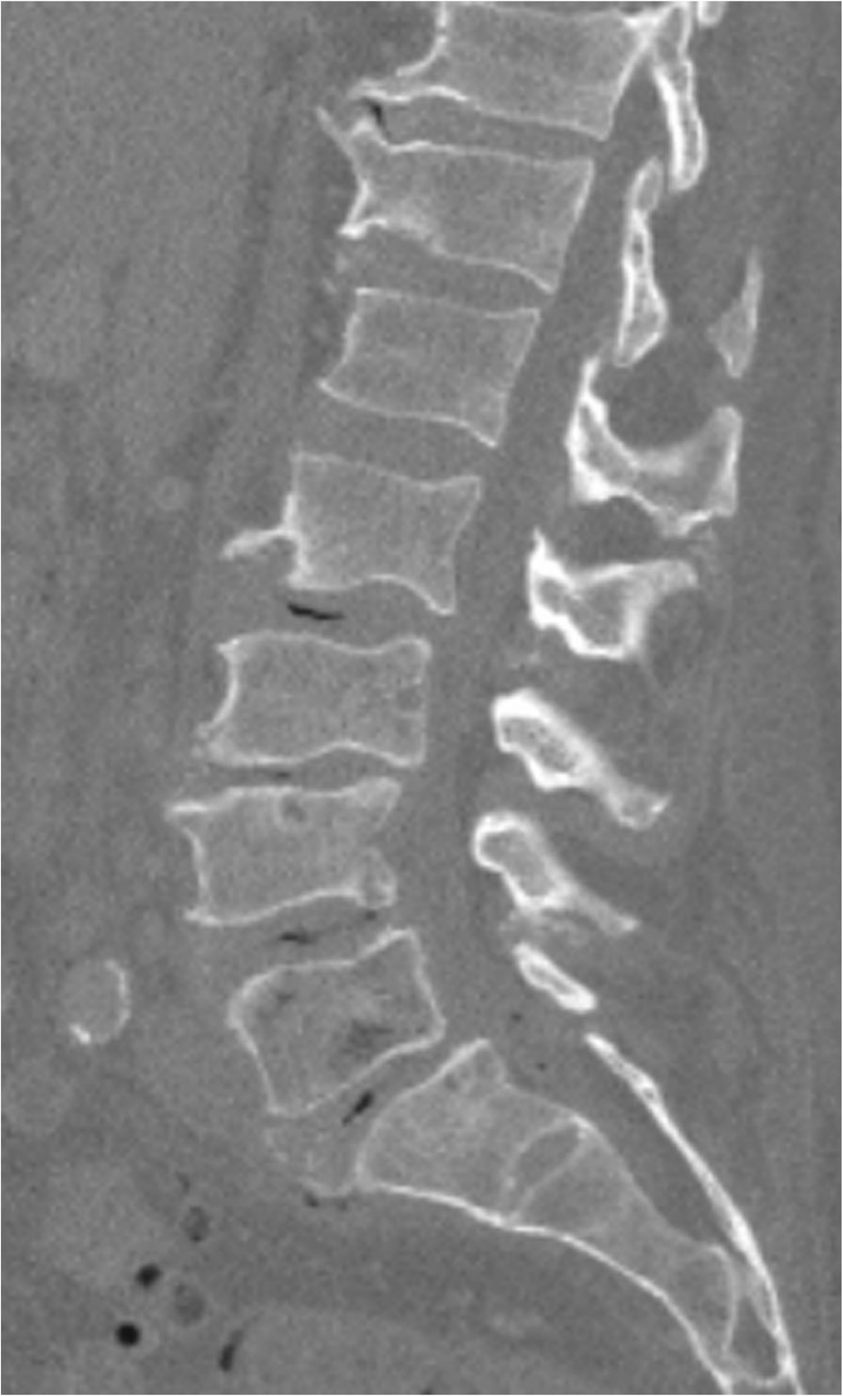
Lumbar CT Sagittal reconstruction: L5 with early osteomyelitic change
